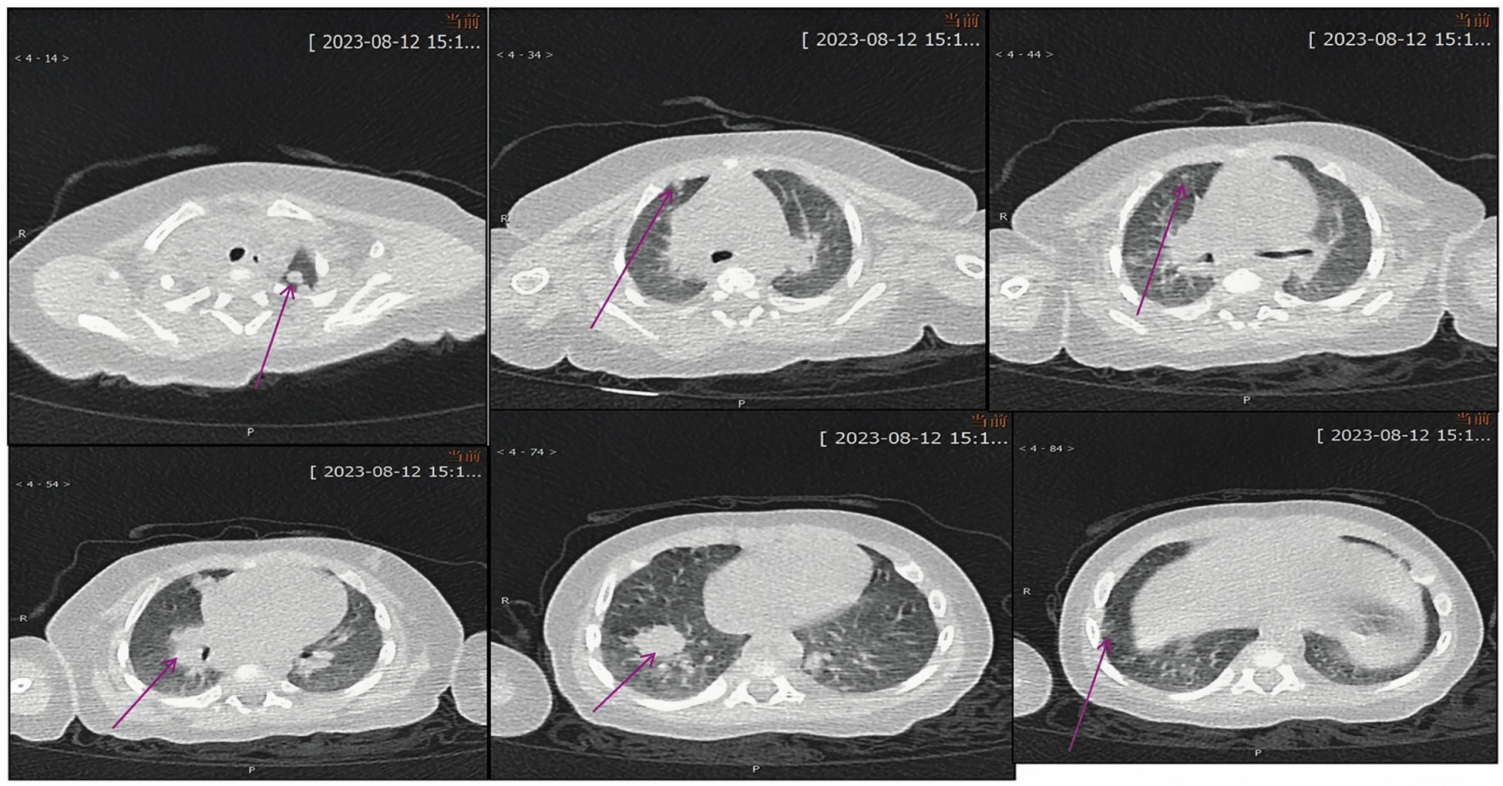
Chest CT prior to first hospital discharge on August 12, 2023 showed partial resolution of pulmonary lesions.
